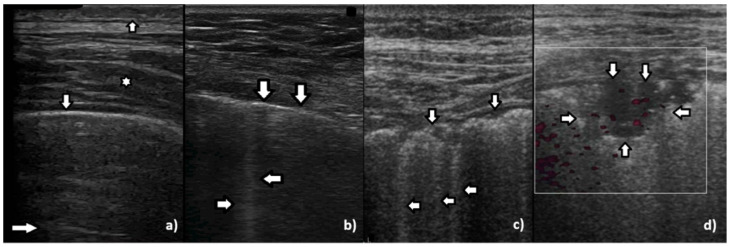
Gradual loss of lung aeration in ultrasound. (a) normally aerated lung, (b) single vertical artifacts, (c) multiple vertical artifacts, and (d) subpleural consolidation.
